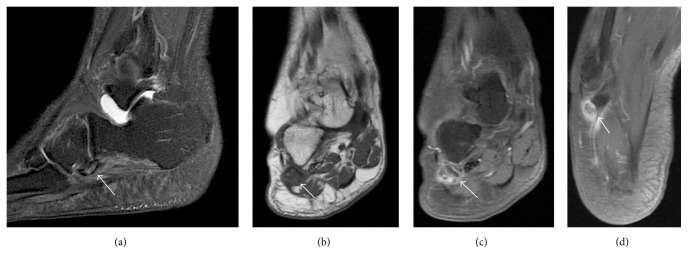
60-year-old female right midfoot MRI. (a) Sagittal T2; (b) coronal T1; (c) fat suppressed T1 postgadolinium; and (d) axial fat suppressed T1 postgadolinium. Arrows show the os peroneum within the peroneal tendon, with irregular contours, bone marrow edema, and intense enhancement of the adjacent soft tissues, characterizing inflammatory changes. The peroneus longus tendon is thickened and heterogeneous, consistent with associated tendinopathy.
